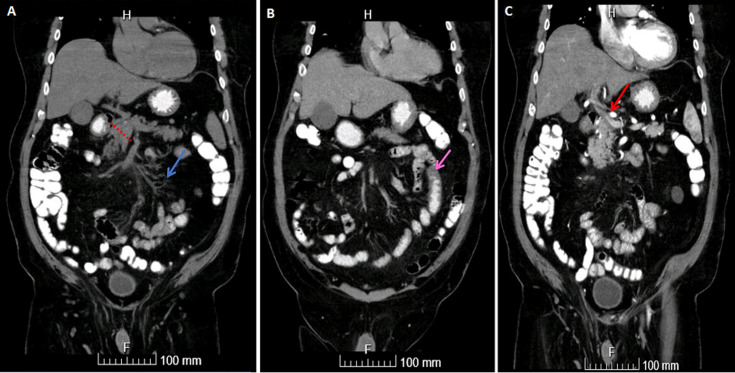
A,B,C) CT of abdomen and pelvis with contrast; the image showing occlusion in SMA (dashed line) and severe stenosis of its distal portion with occlusion of jejunal branches (blue arrow); a jejunal loop showing wall thickening (pink arrow) and submucosal oedema; stenosis of the celiac artery (red arrow)
